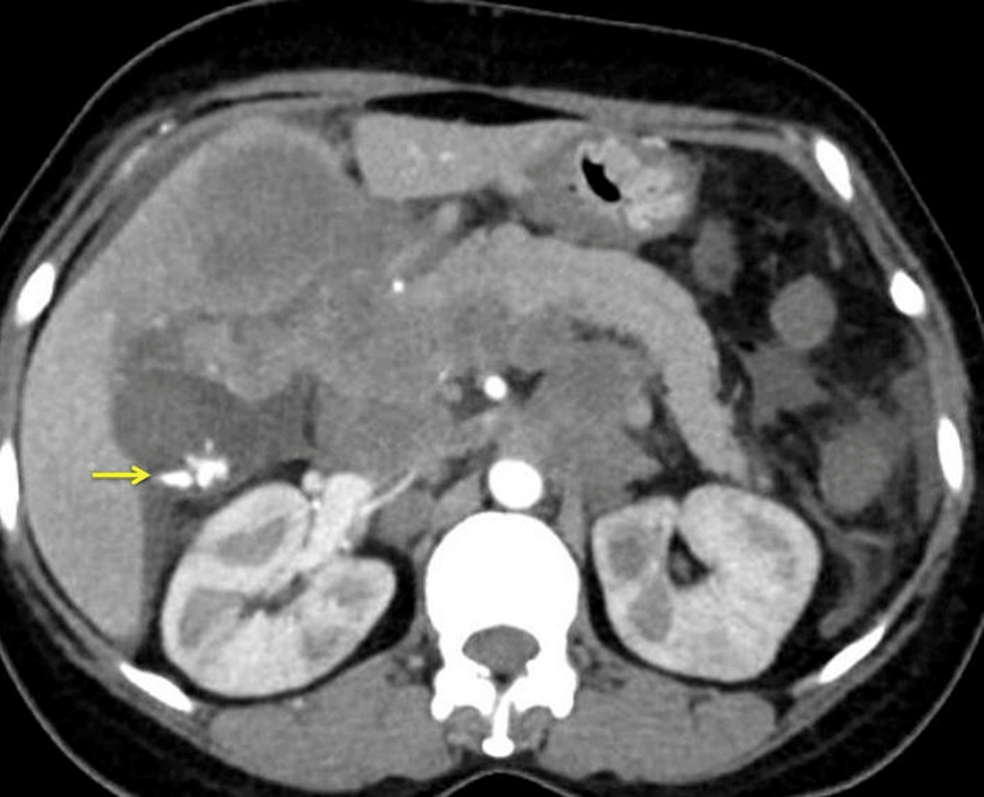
CT of the abdomen showing cholelithiasis (arrow)
